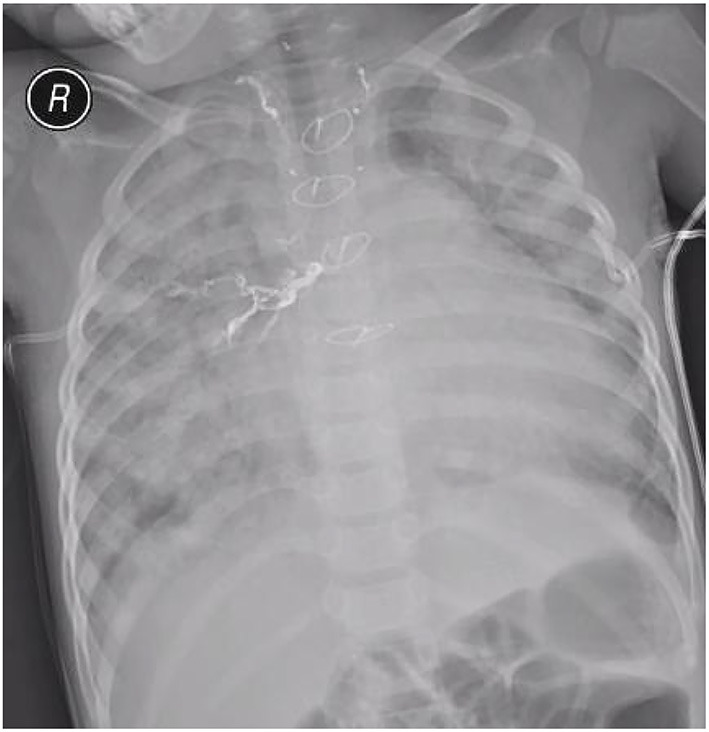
Post-operative chest X-ray showing a representative image of severe pulmonary infection.
